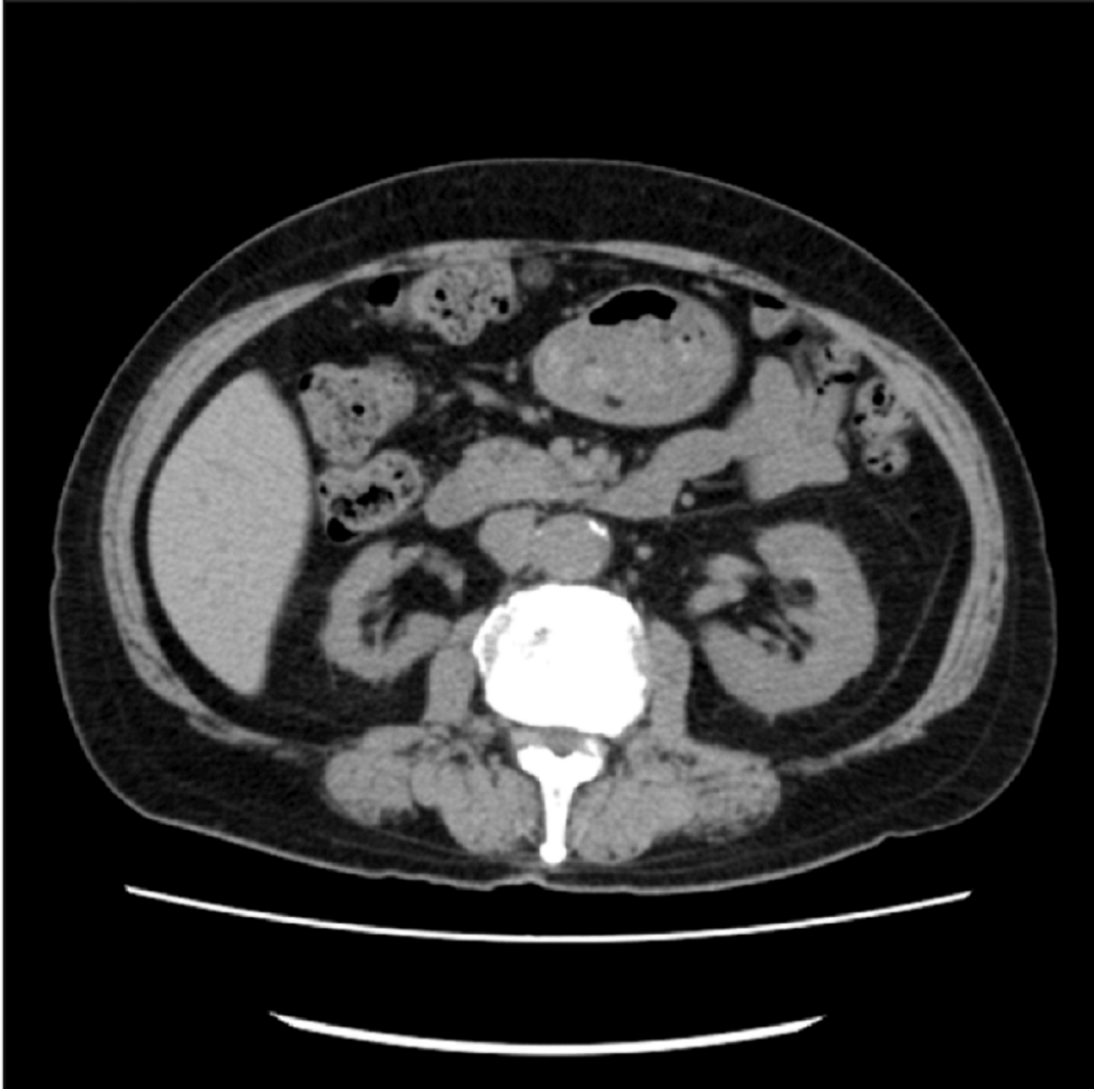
Metastatic sites in patient 3 Computed tomography imaging revealed no apparent visceral metastases.
